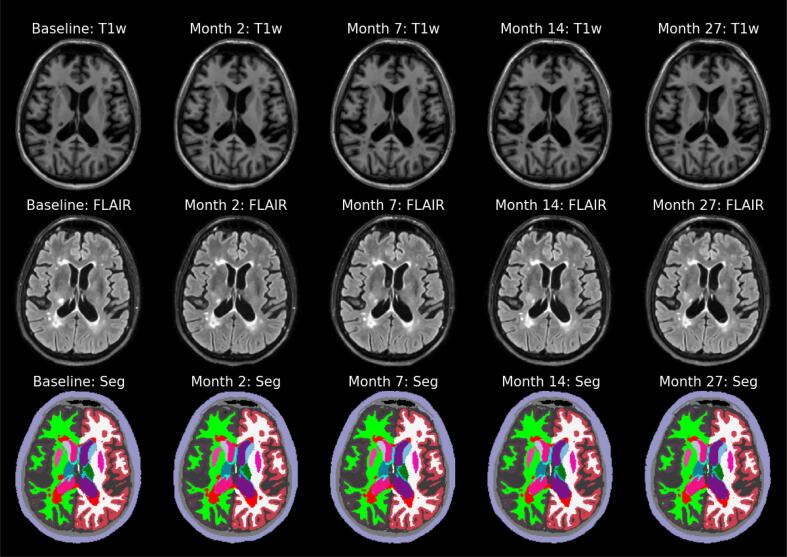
Whole-brain and white matter lesion segmentations (labeled in red) produced by the proposed method from T1w and FLAIR longitudinal scans of an MS patient. T1w=T1-weighted, FLAIR=FLuid Attenuation Inversion Recovery, MS=Multiple Sclerosis.
